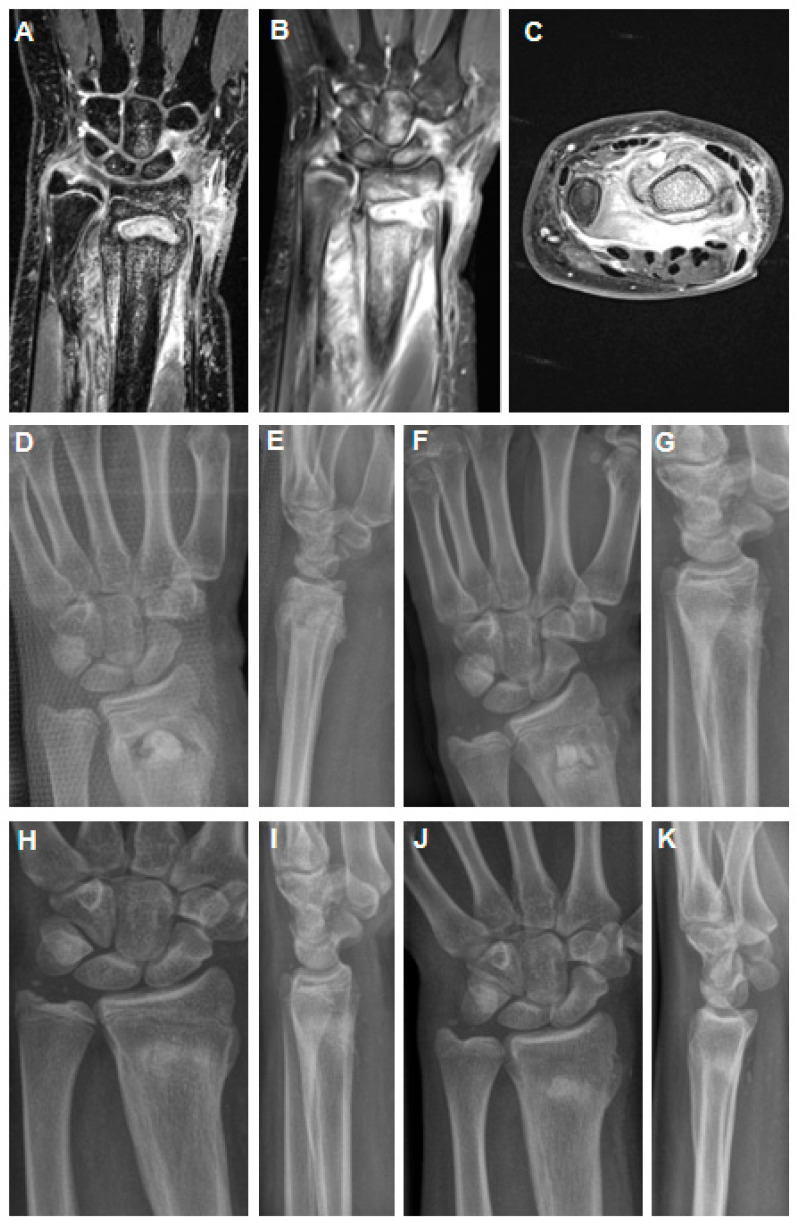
MRI imaging 4 weeks after K-wire removal after initial closed reduction and K-wire fixation of a dislocated distal radius fracture in an 11-year-old girl (A–C). Abscess formation in the distal metaphysis of the radius with intensive periosteal reaction (A,B) and affection of the growth through the K-wire channel. Axial section revealed strong abscess into the palmar soft tissues (C). Post-operative X-rays show filling of the metaphyseal defect area with a PMMA spacer (D,E). The spacer was exchanged against a hydroxyapatite–calcium sulphate biomaterial 9 weeks after stage 1 (F,G). After 6 weeks, there was already good remodeling of the metaphyseal bone zone (H,I). At midterm follow-up of 16 months, there was correct alignment of the wrist joint with premature closure of the growth plate (J,K).
